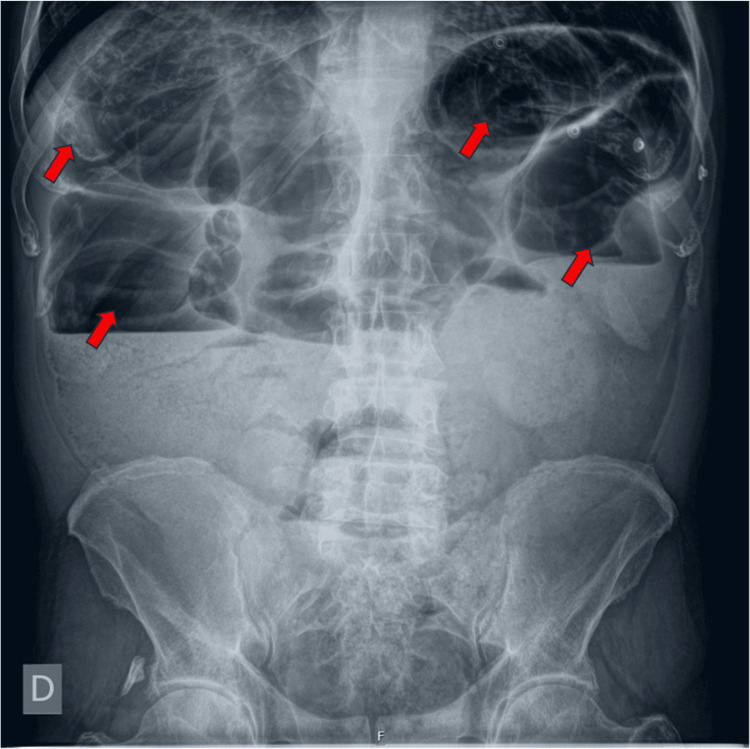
Abdominal radiograph depicting multiple air-fluid levels (red arrows) D – right side of the patient's body, F – foot end of the patient's body
